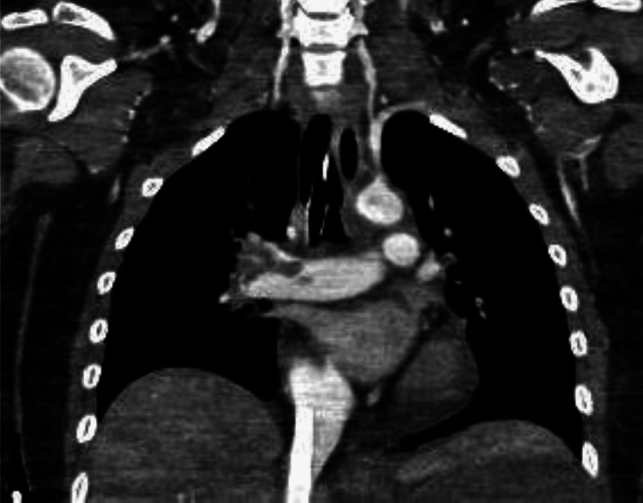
Coronal CTA scan that reveals massive arterial occlusion with failure to enhance the entire lumen due to a substantial filling defect of the right lobar arteries
